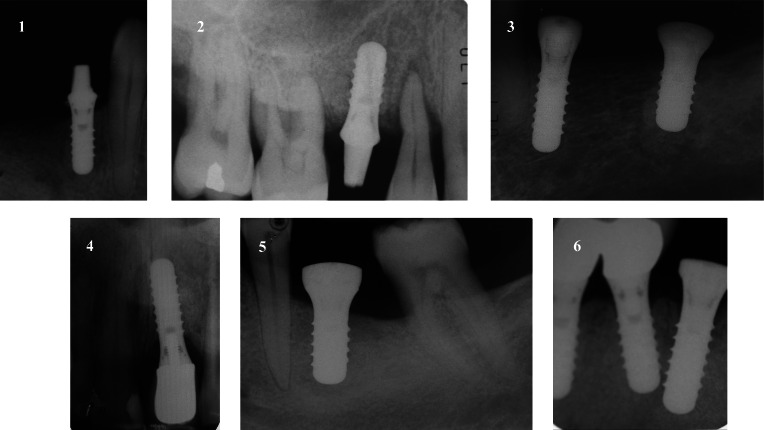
The six implants at a radiographic examination three months after surgical treatment of RPI
